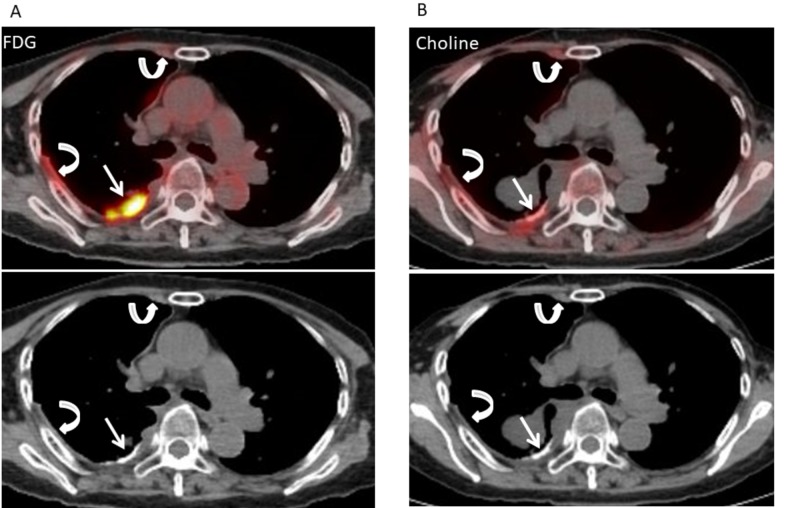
Fluorodeoxyglucose (FDG) and 11C-choline positron emission tomography/computed tomography (PET/CT) after talc pleurodesis and neoadjuvant chemotherapy (NAC) for patient 2. A. Axial fluorodeoxyglucose (FDG)-positron emission tomography/computed tomography (PET/CT) after talc pleurodesis and NAC shows intense FDG uptake (maximum standardized uptake value, 5.74) in the high attenuation areas of right pleural thickening (arrow) and mild FDG uptakes (maximum standardized uptake value, 2.47) in the non-high attenuation areas of right pleural thickening (arrows). B. Axial 11C-choline PET/CT after talc pleurodesis and NAC shows mild choline uptake (maximum standardized uptake value, 2.97) in the high attenuation areas of right pleural thickening (arrow) and faint choline uptakes (maximum standardized uptake value, 2.19) in the non-high attenuation areas of right pleural thickening (arrows).
